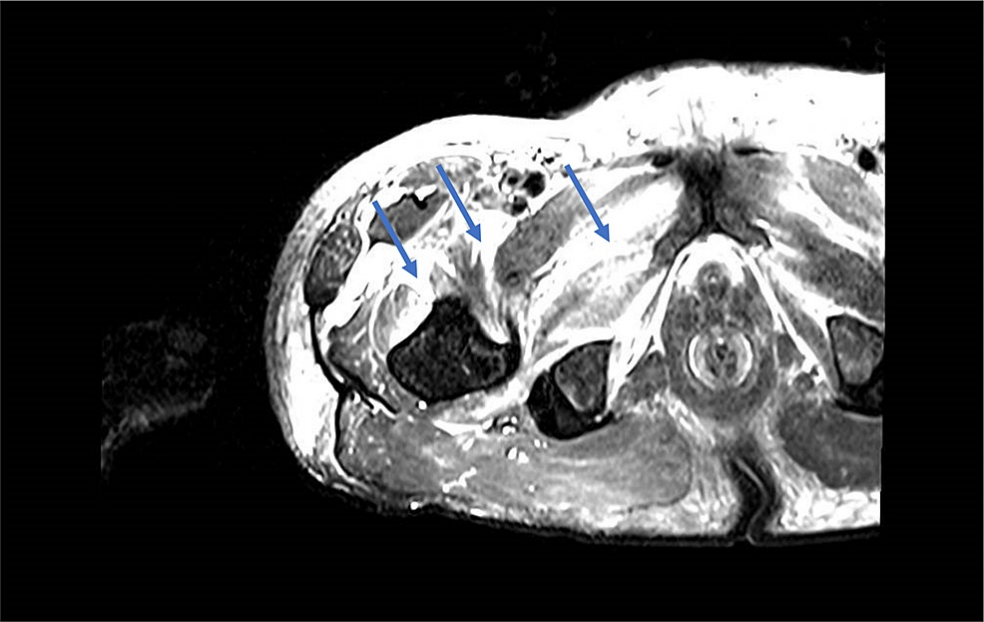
Axial MRI demonstrating increased volume and heterogeneity of the adductor, vastus and sartorius muscles (arrows) associated with perifascial edema and multiple areas compatible with muscle infarction. MRI, magnetic resonance imaging
